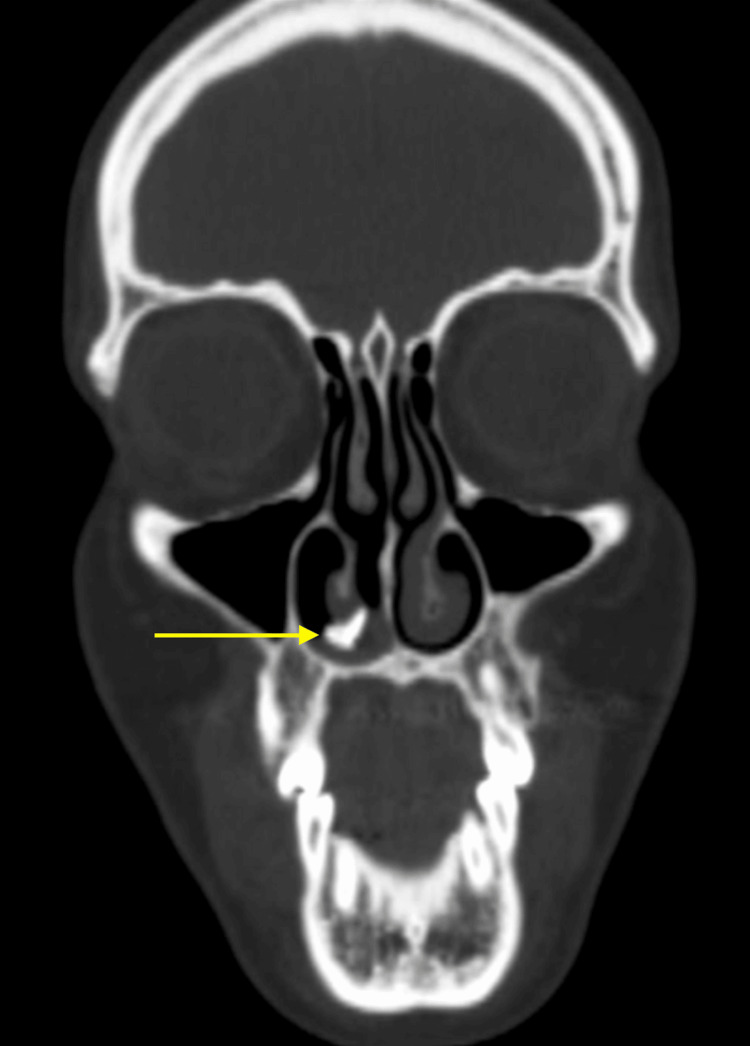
Non-contrast CT scan of the paranasal sinuses demonstrating a well-defined hyperdense calcified lesion along the floor of the right nasal cavity (arrow), consistent with a rhinolith.
